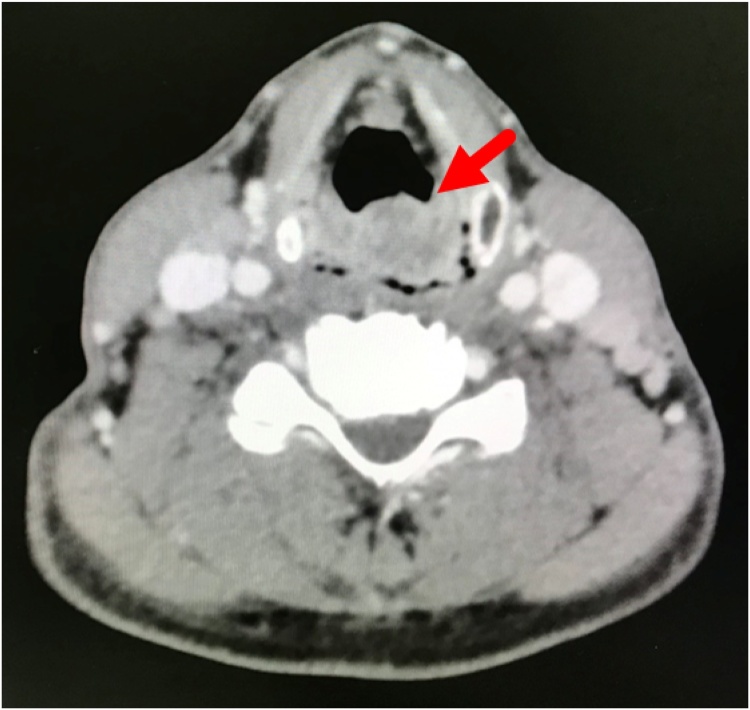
Axial view of contrasted CT showed heterogenous irregular dense mass at anterior wall of hypopharynx with extension anterior to both arytenoid cartilage, posterior commissure, posterior aspect of true vocal cords, subglottic, and pyriform sinuses (red arrow).
